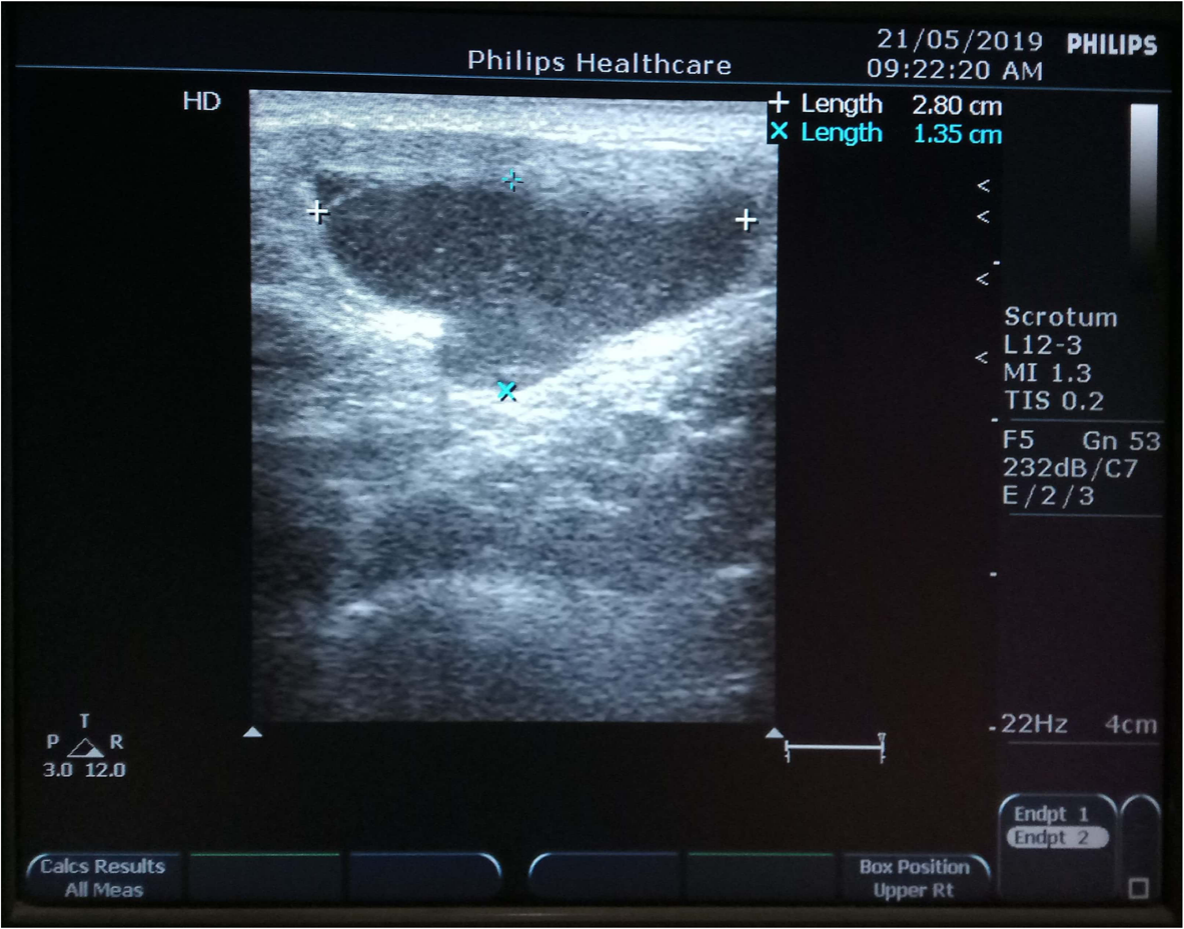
Grayscale ultrasonography of the right inguino-labial region reveals a mildly echogenic cystic swelling of size 2.80 cm × 1.35 cm below the inguinal ligament and medial to the femoral vessels without any intra-abdominal communication
